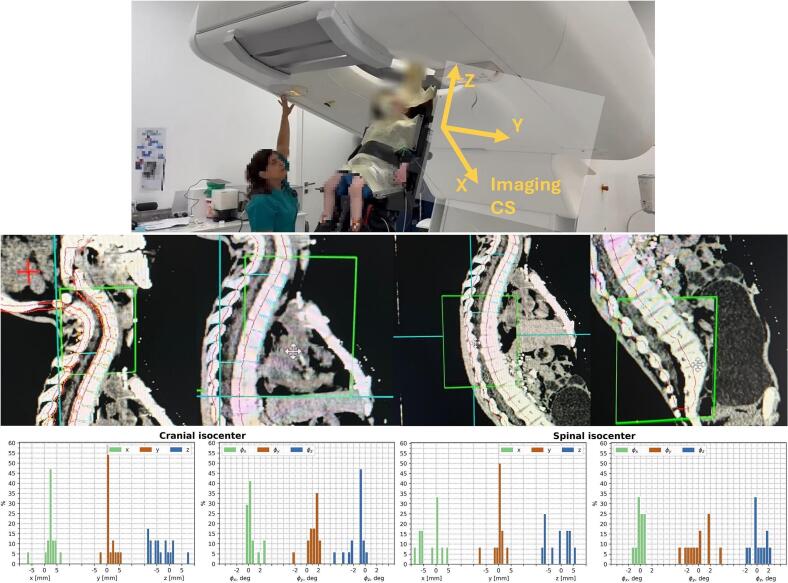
Patient setup for daily 3D/3D registration using the vertical CT scanner (top panel). Example of patient position verification prior to treatment across different regions of the craniospinal axis (middle panel). The area within the green box represents the daily CT data, while the surrounding area shows the reference planning CT. Results of daily 3D/3D image registration for the cranial and spinal isocenters are shown separately (bottom panel). (For interpretation of the references to colour in this figure legend, the reader is referred to the web version of this article.)
